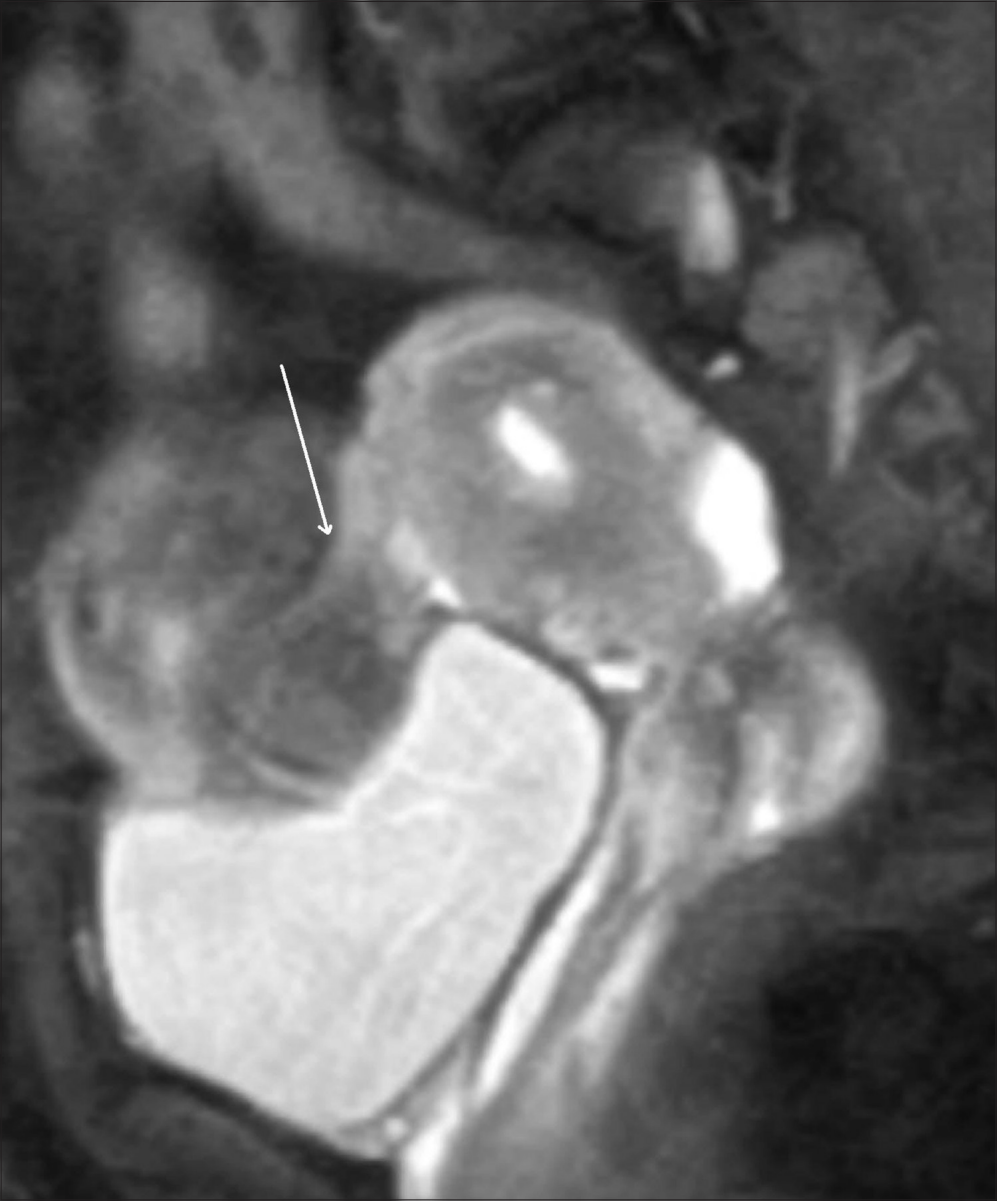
Subserosal fibroid. Sagittal fat-suppressed T2W MRI image shows a pedunculated subserosal fibroid along the anterior wall (white arrow shows the stalk)
